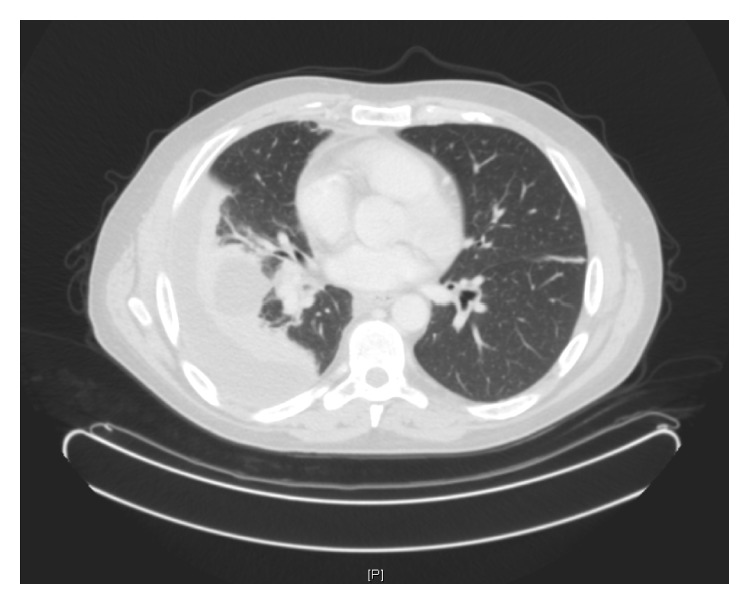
Computed tomography scan showing right-sided middle and lower lobe pulmonary consolidations with a moderately sized right-sided pleural effusion. There was no evidence of underlying malignancy.
